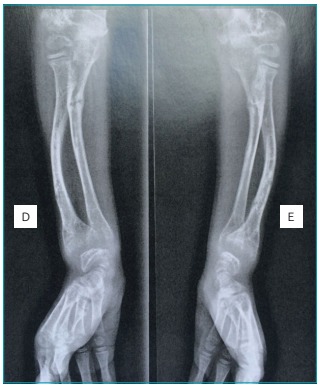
Forearm x-ray after the beginning of therapy.
